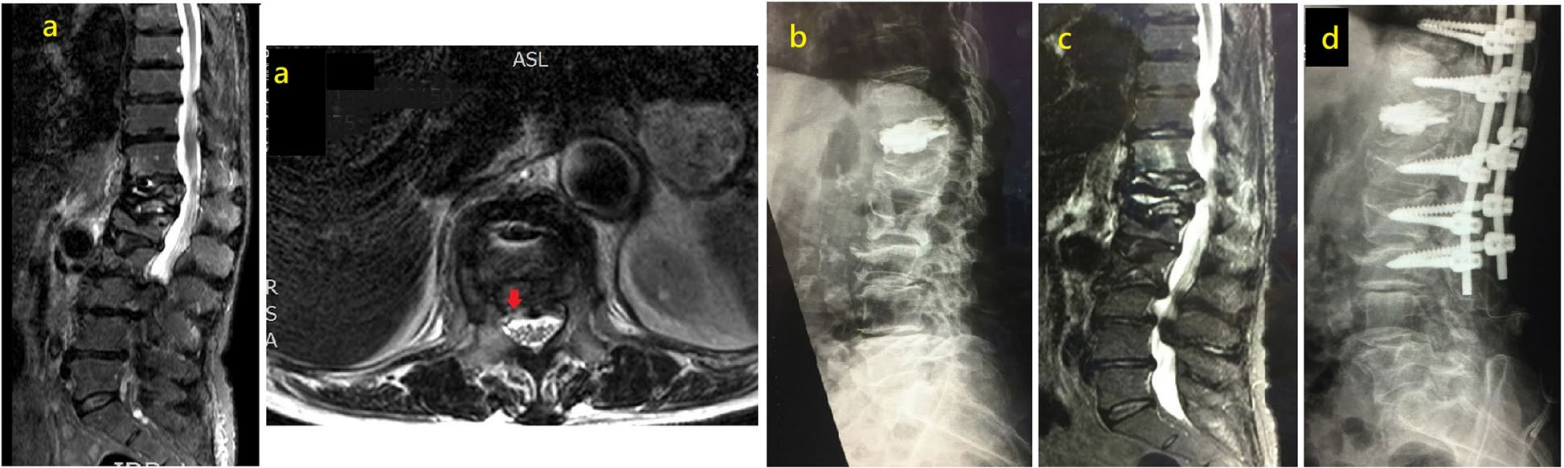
MRI showing a 77-year-old female with T12 compression fracture, disrupted posterior vertebral rim was identified (red arrow) (a). Lateral view of lumbar spine X-ray of a T12 VCF underwent PKP with 8.0 c.c. bone cement (b). Patient developed right T12 radiculopathy 2 months after PKP, MRI showing spinal canal compromised by a retropulsed bone fragment (c). We performed posterior decompression (PD) of T12 with instrumentation from T10-L3 (d)
